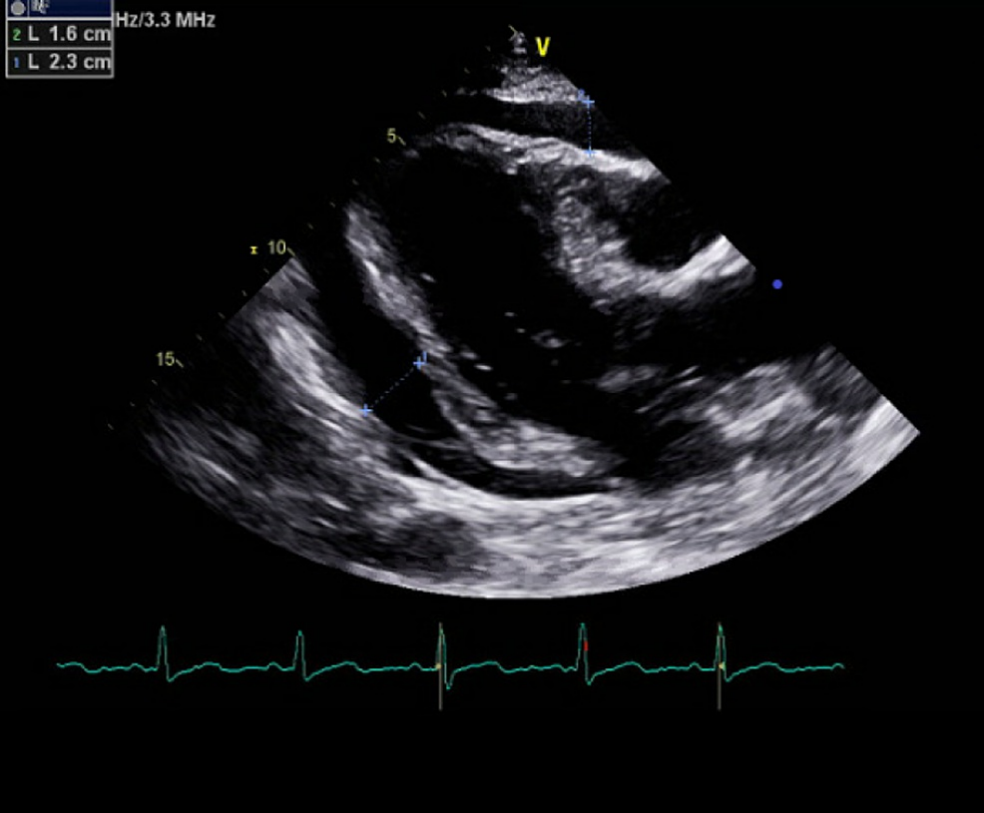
Echocardiogram showing large pericardial effusion
